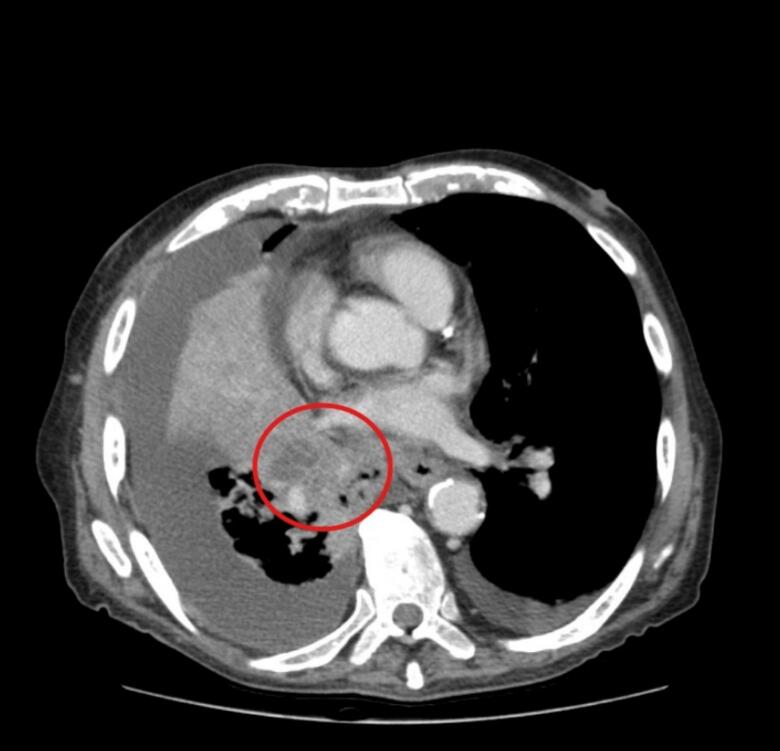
Axial CT scan with IV contrast showed ill-defined infiltrative hypodense right hilar perihilar lesion.
